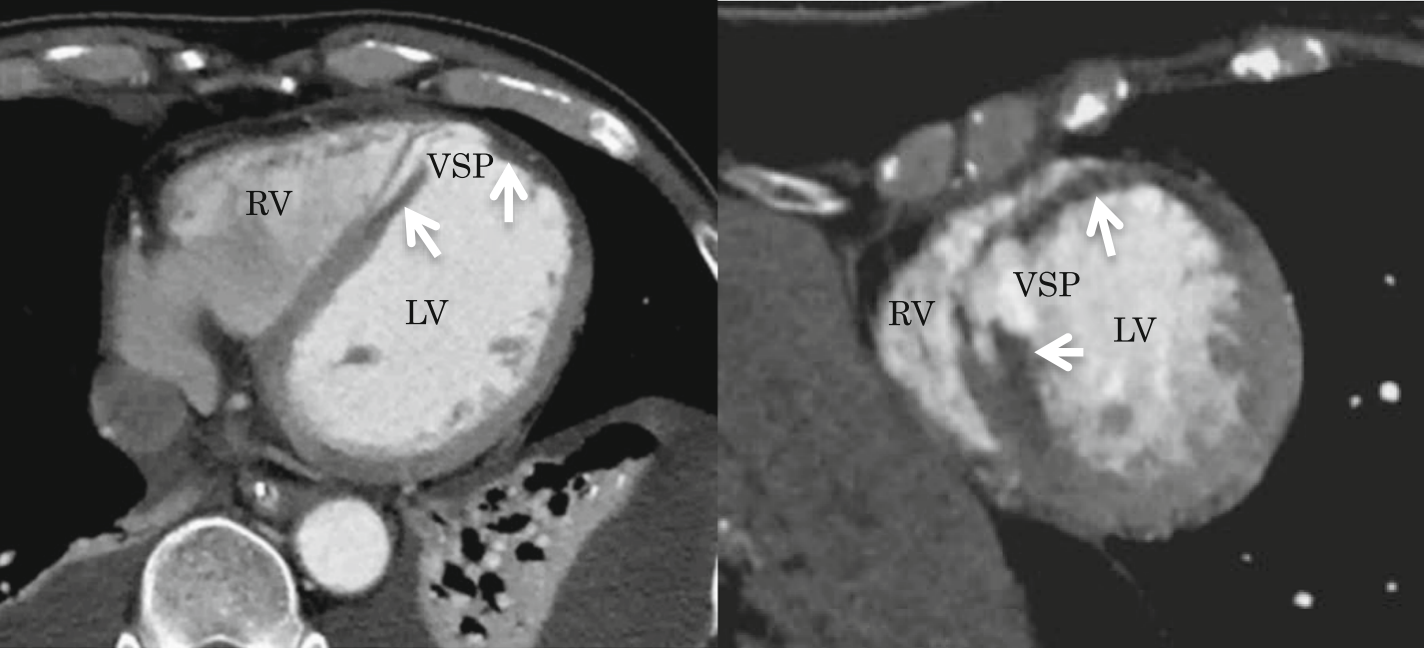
Preoperative computed tomography shows the myocardium having poor contrasting that became light thickness (white arrows)
